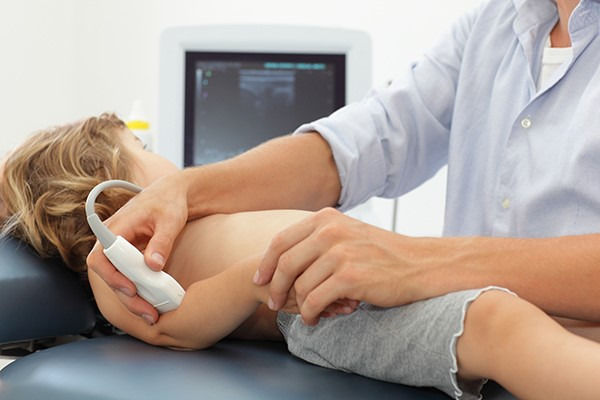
Transducer position to obtain views to confirm pulled elbow. In this image the ultrasound probe is orientated caudally.
